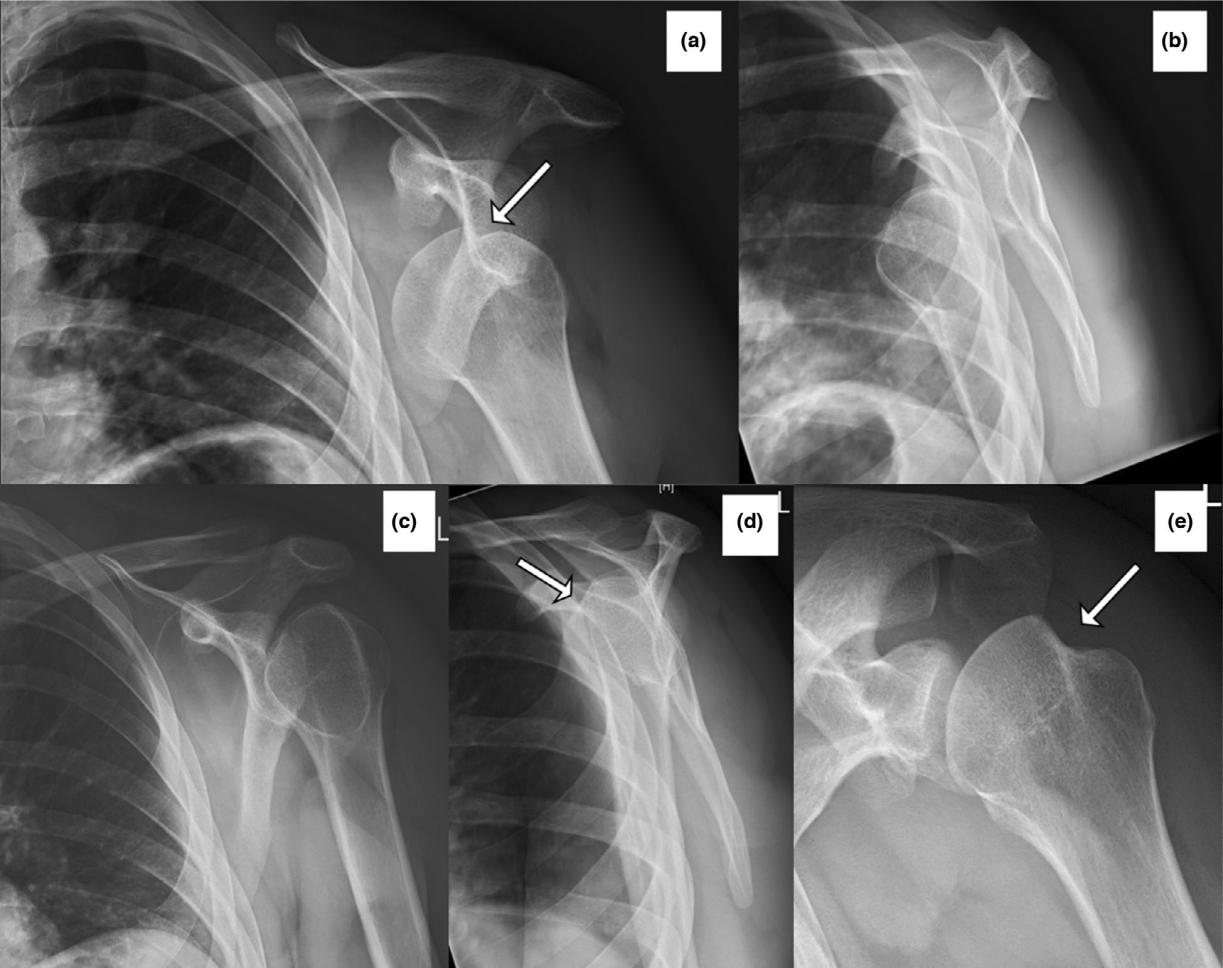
The patient presented with a dislocation to rule out fractures. Radiographs were taken pre (A‐B) and post (C‐E) shoulder reduction. AP shoulder (A) and lateral scapula shoulder (B) show anterior dislocation and slight indentation of the superior humeral head suggestive of a Hill‐Sachs defect. The post‐reduction AP (C), lateral scapula (D), and MTA (E) view show indentation and sclerosis in the superior humeral head. The Hill‐Sachs lesion (arrow) is best demonstrated on the MTA view (E). MTA, Modified trauma axial.
